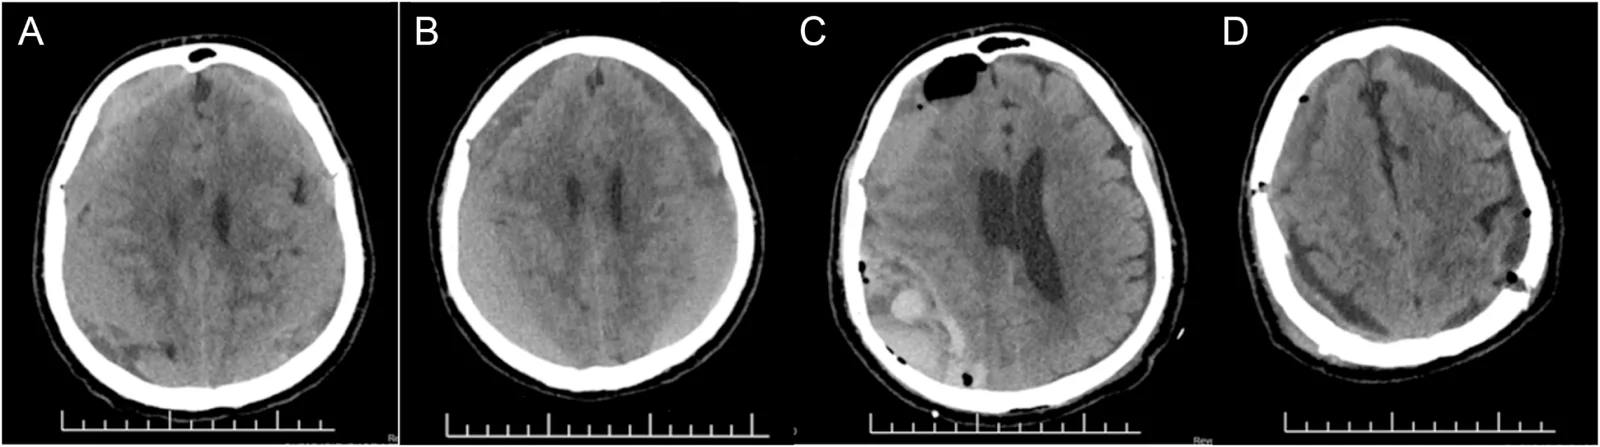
(A) Bilateral acute SDH with subacute components; (B) CT scan on the following day showing progressive hemorrhage; (C) Rebleeding area in the early post-operative period; (D) Pre-discharge imaging.
